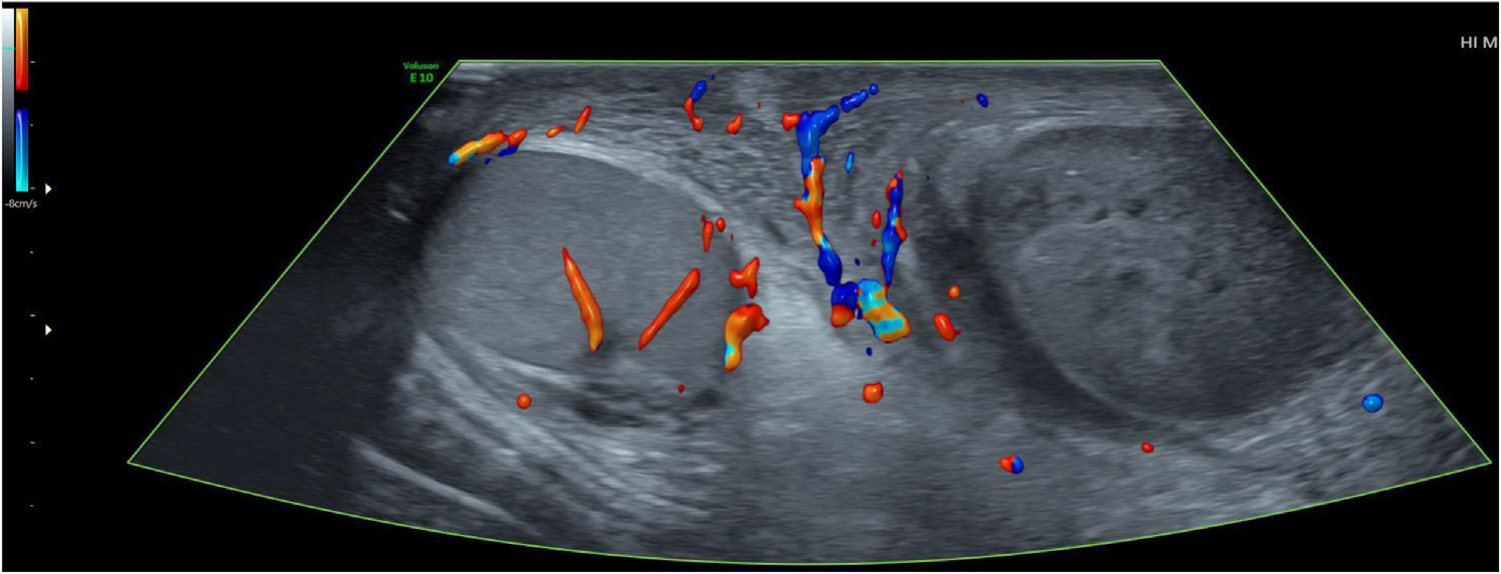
Comparative color Doppler study of the testicles: demonstrates the absence of vascularization of the left testicle. We also note the hyperhemia and thickening of scrotal wall.
